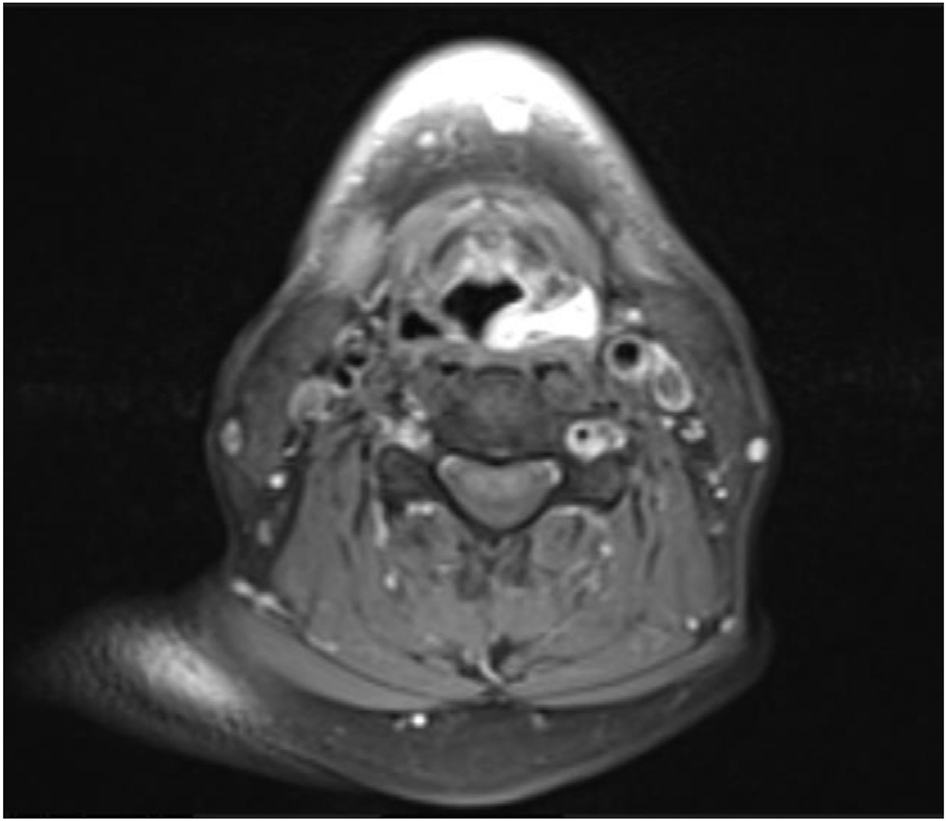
Magnetic resonance imaging with contrast of the left piriform fossa, showing a hypointense T2 signal with intense post-contrast enhancement.
